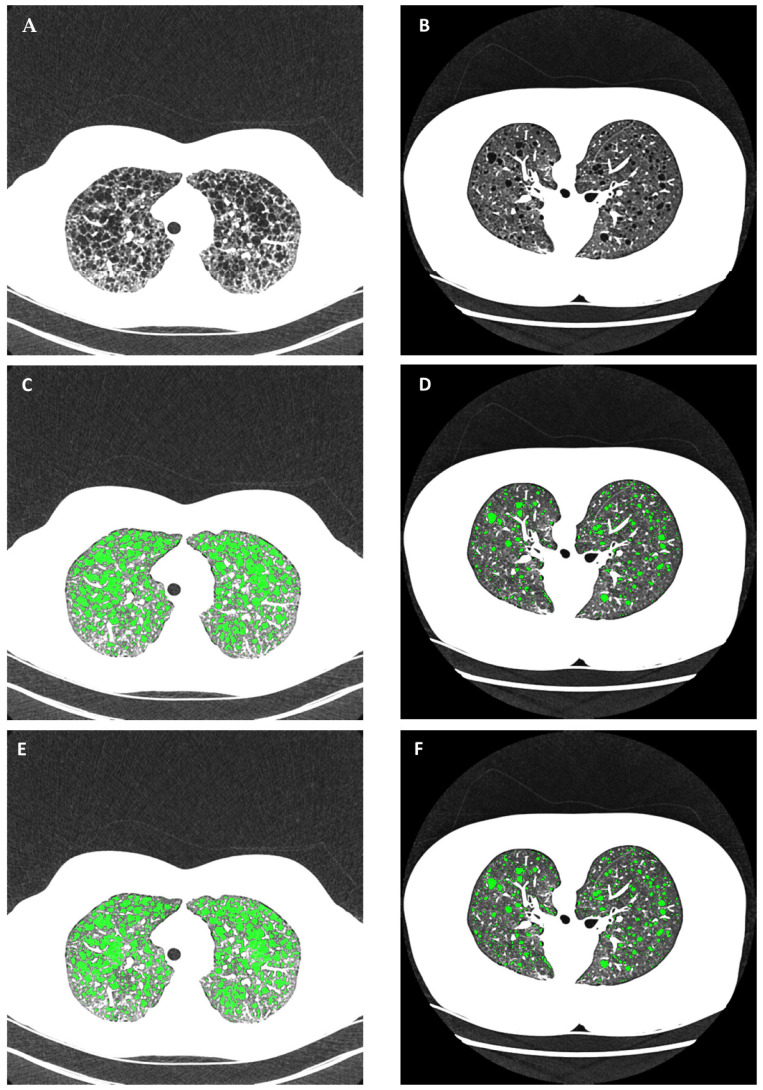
An example of the variability in the radiodensity of air and non-cystic lung parenchyma across different patients and scanner platforms and the consequences on cyst segmentation. (A,B) Chest HRCT scans of two patients from two different scanner platforms in supine and prone positions, respectively. Both are displayed in the grayscale range of −1050 HU to −600 HU to highlight lung parenchymal structures. The average radiodensity of the air background outside the body was −971 HU in (A) and −1006 HU in (B). The average radiodensity of non-cystic lung parenchyma was −805 HU in (A) and −918 HU in (B). (C,D) Optimal cyst segmentation was determined by a trained operator using the gold-standard commercial semi-automated software. The threshold radiodensity for cystic areas was adjusted by the operator to −880 HU for the image series in (A) and −970 HU for the image series in (B). The cystic areas are highlighted in green. (E,F) Automated cyst segmentation without user input. The automated method accounted for the variability of radiodensity values to provide the appropriate segmentation (highlighted in green).
